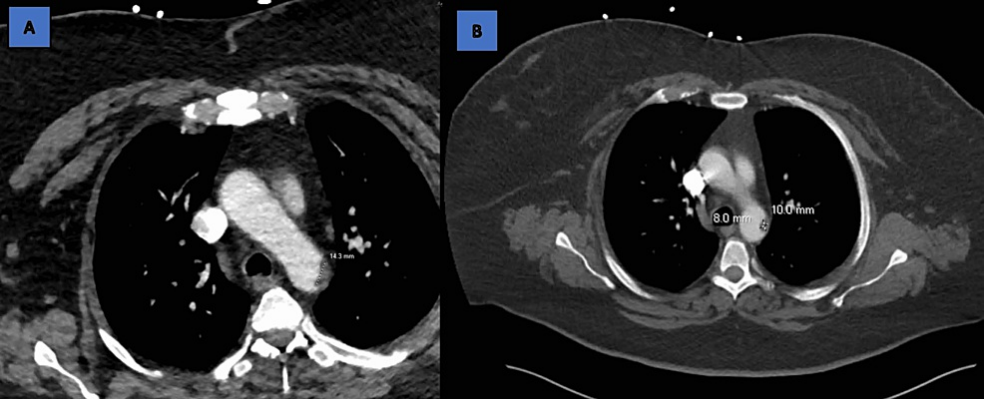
A. CTA head and neck shows filling defect at the aortic isthmus measuring 1.4 cm, unchanged from the previous. Consider focal noncalcified plaque or mural thrombus. B. CT aortic dissection protocol. The prior imaging in January 2023 that demonstrates focal distal aortic arch/proximal descending aortic plaque 1.0x0.8x1.1 cm. CTA: computed tomography angiogram
